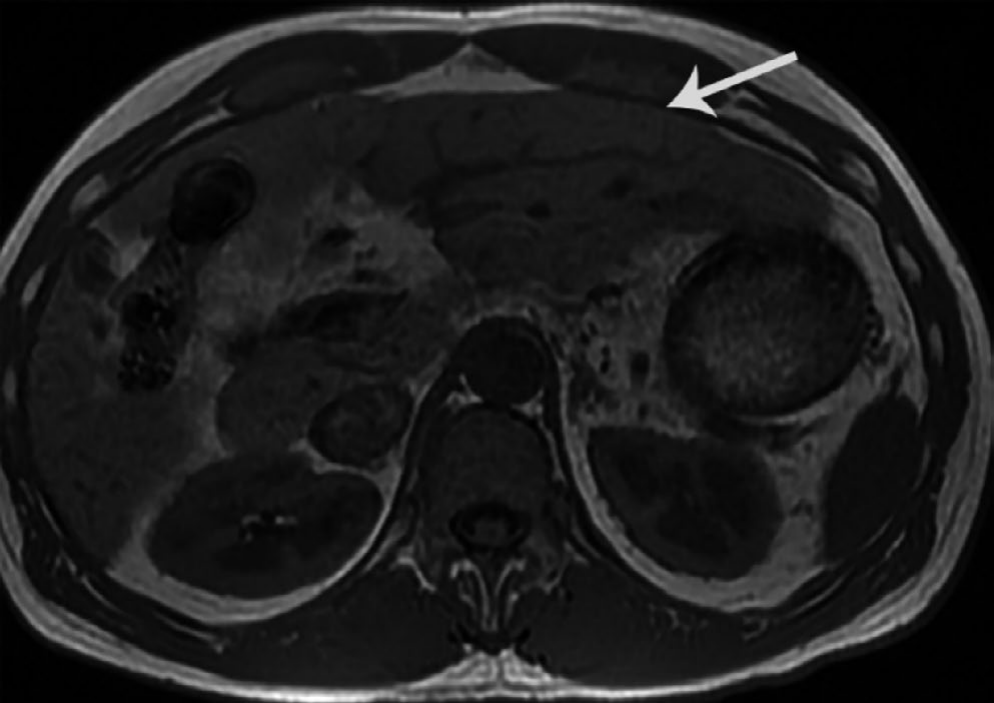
Hepatic surface nodularity, MRI
